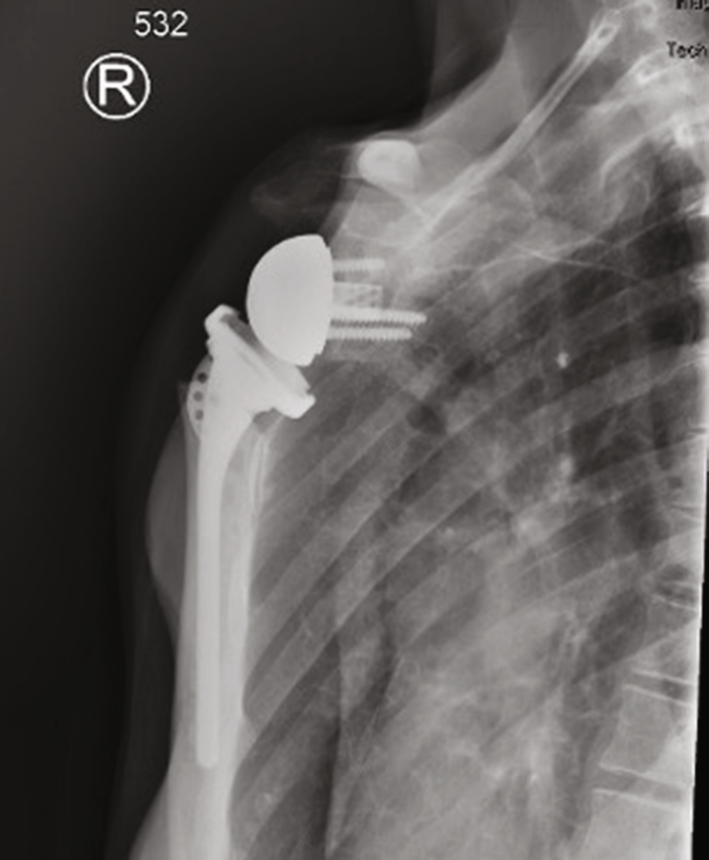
A 1-week postoperative AP radiograph showing appropriate alignment and fixation of the right reverse total shoulder arthroplasty components.
